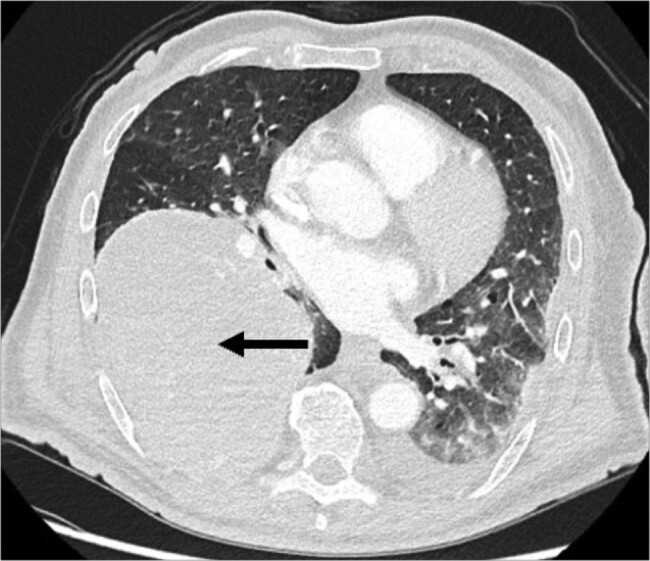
Computed tomography scan. A large solid mass of the right pleura with a diameter of 14 × 14 × 11 cm located above the diaphragm.
